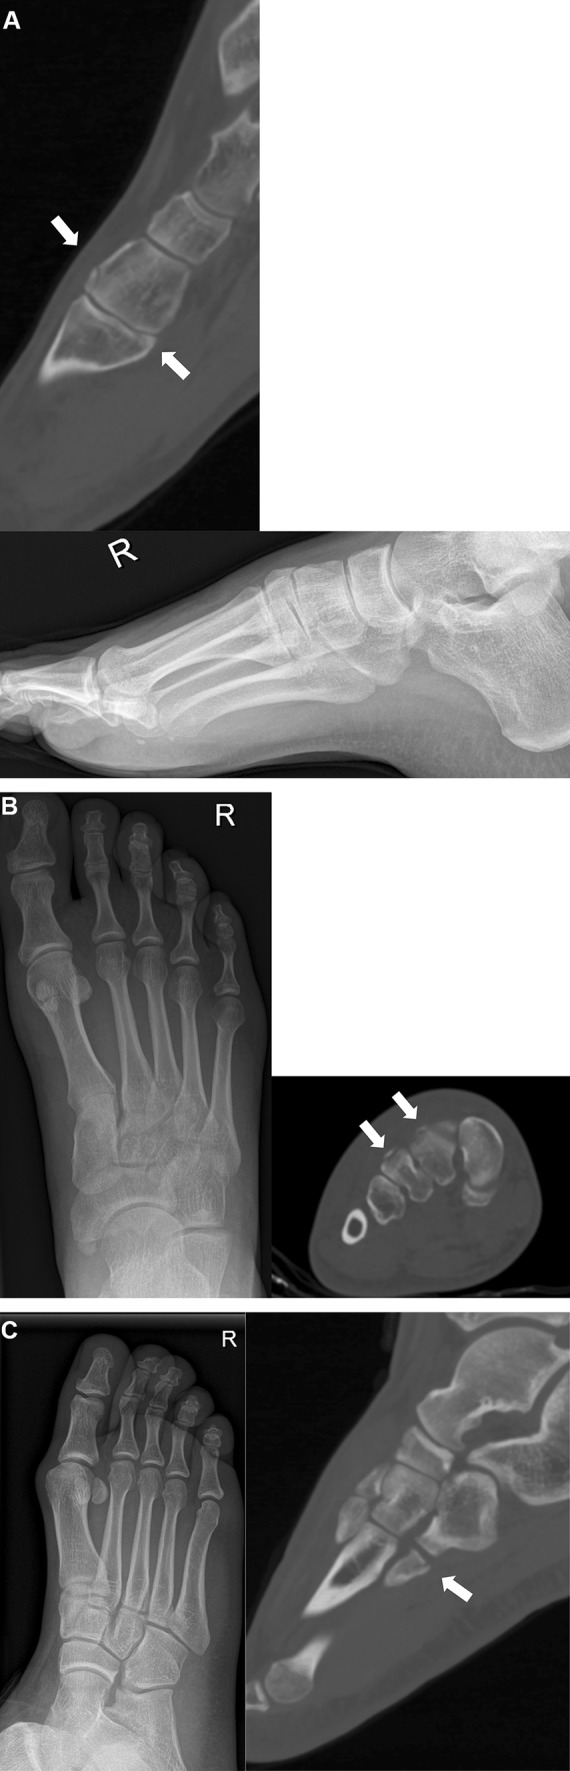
a–c Radiological findings of the three undisplaced injuries which were missed by all observers. a No specific findings with standard radiographs, yet CT revealed fractures of the first metatarsal base and medial cuneiform. b No specific findings with standard radiographs, yet avulsion fractures of the second, third and the fourth metatarsal bases were detected in CT. c No specific findings with standard radiographs, yet avulsion fractures of the fourth metatarsal base were detected in CT
